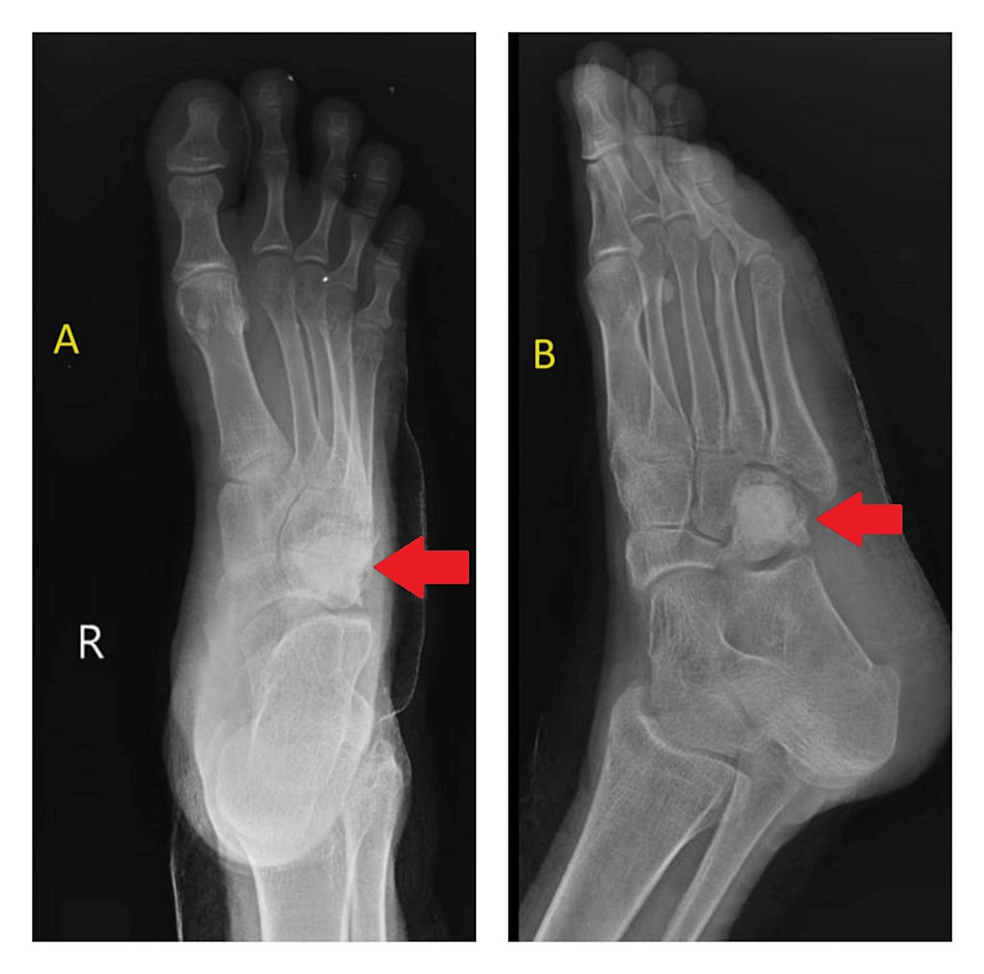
Post-operative X-ray of the right foot in anteroposterior (A) and oblique (B) views showing that the cuboid bone was filled with bone cement, the infection was resolved, and the cuboid lesion was consolidated
